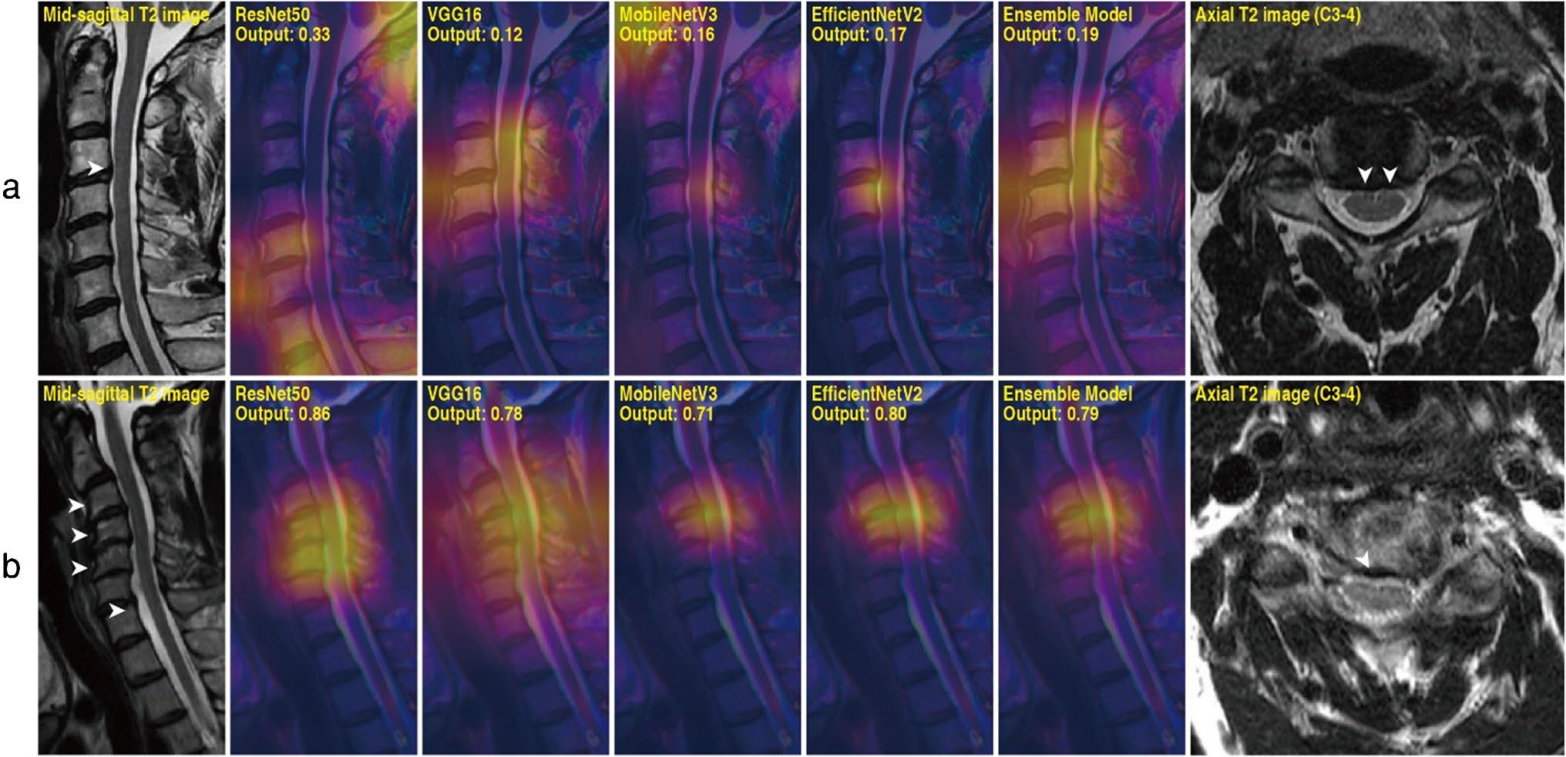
Grad-CAM results of test samples producing false predictions. Non-contrast mid-sagittal T2-weighted MRI image is shown, followed by a series of Grad-CAMs obtained from different models and an axial section of the most affected region. a A 62-year-old male of Kang grade 2 with spinal cord compression at C3-4 (arrowheads). b A 48-year-old female of Kang grade 1 with severely progressed cervical spondylosis at C3-5 (arrowheads)
